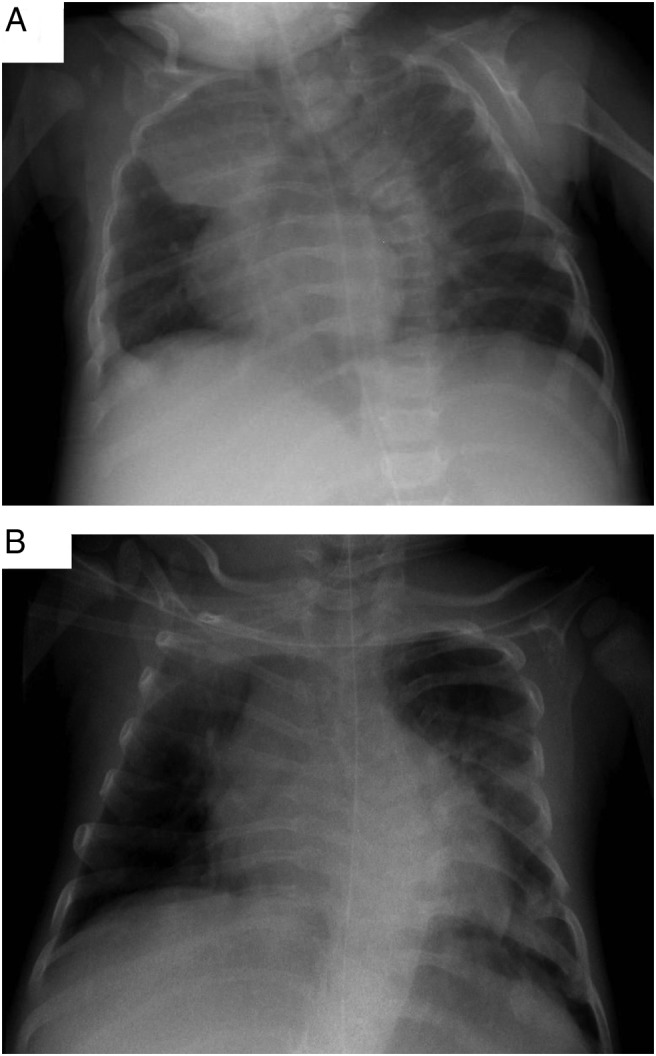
A, Anteroposterior chest x-ray of patient 2 performed on day 101 demonstrating thoracolumbar scoliosis and left rib fractures located on ribs 7, 8, and 9. B, Anteroposterior chest x-rays performed on day 412 demonstrating worsening thoracolumbar scoliosis and persistence of callus formation in left ribs 7, 8 and 9 with a new fracture at left rib 6.
